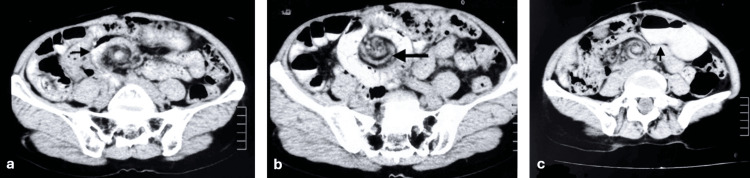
CECT abdomen showing (a) a swirl sign suggestive of midgut volvulus, (b) twisting of the mesenteric vessels, and (c) dilated proximal bowel loops with air-fluid levels CECT: contrast-enhanced computed tomography
